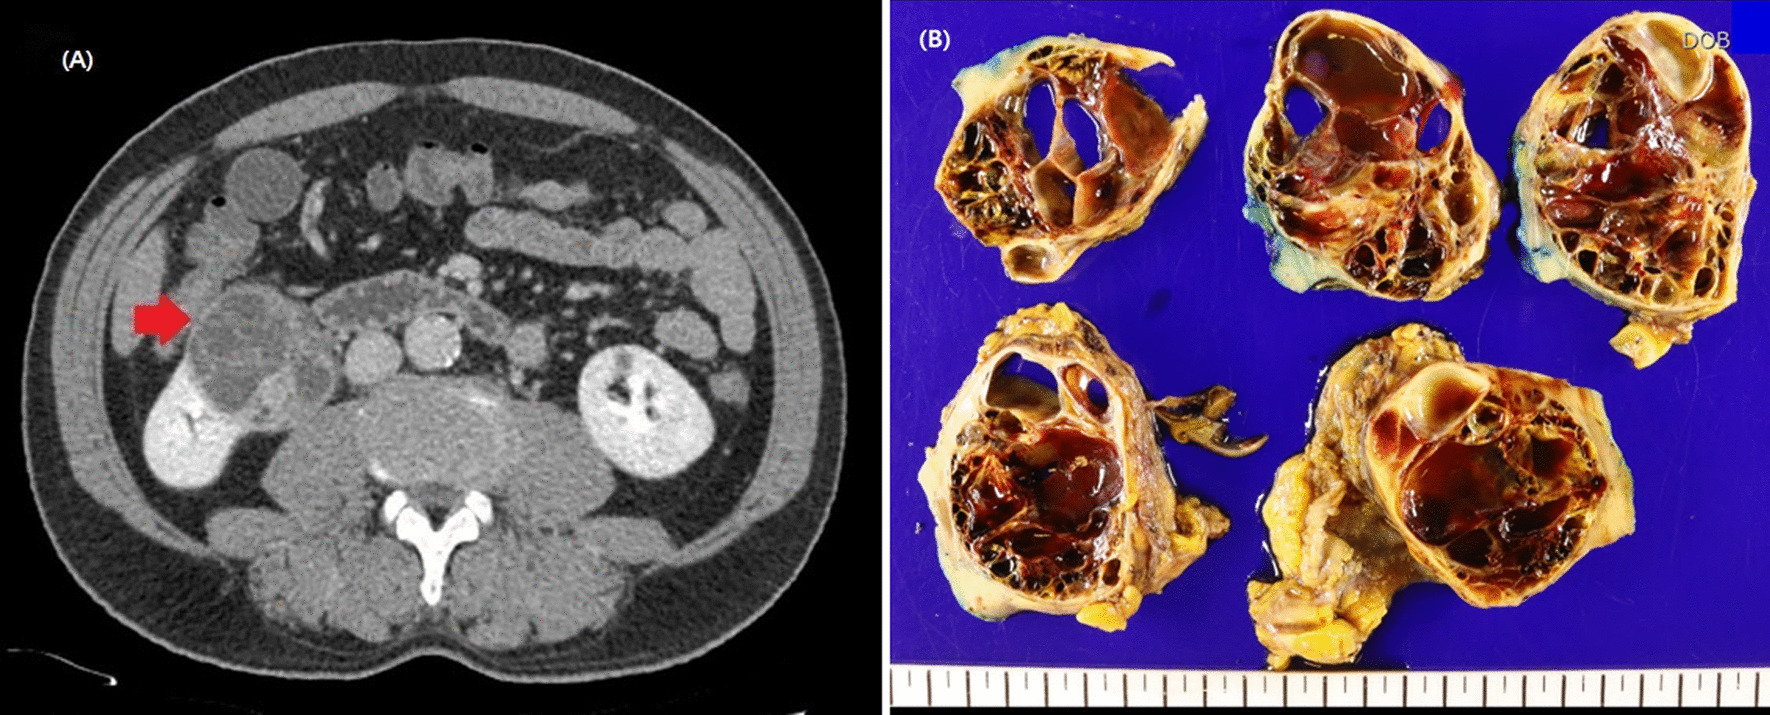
Preoperative contract CT scans of cystic renal tumor and gross picture of retrieved cystic renal cell carcinoma. A Bosniak IV renal cyst showing multi-septated cystic mass with enhancing nodular walls. B Bosniak IV renal cyst showing gross nodular lesion with multiple septation
